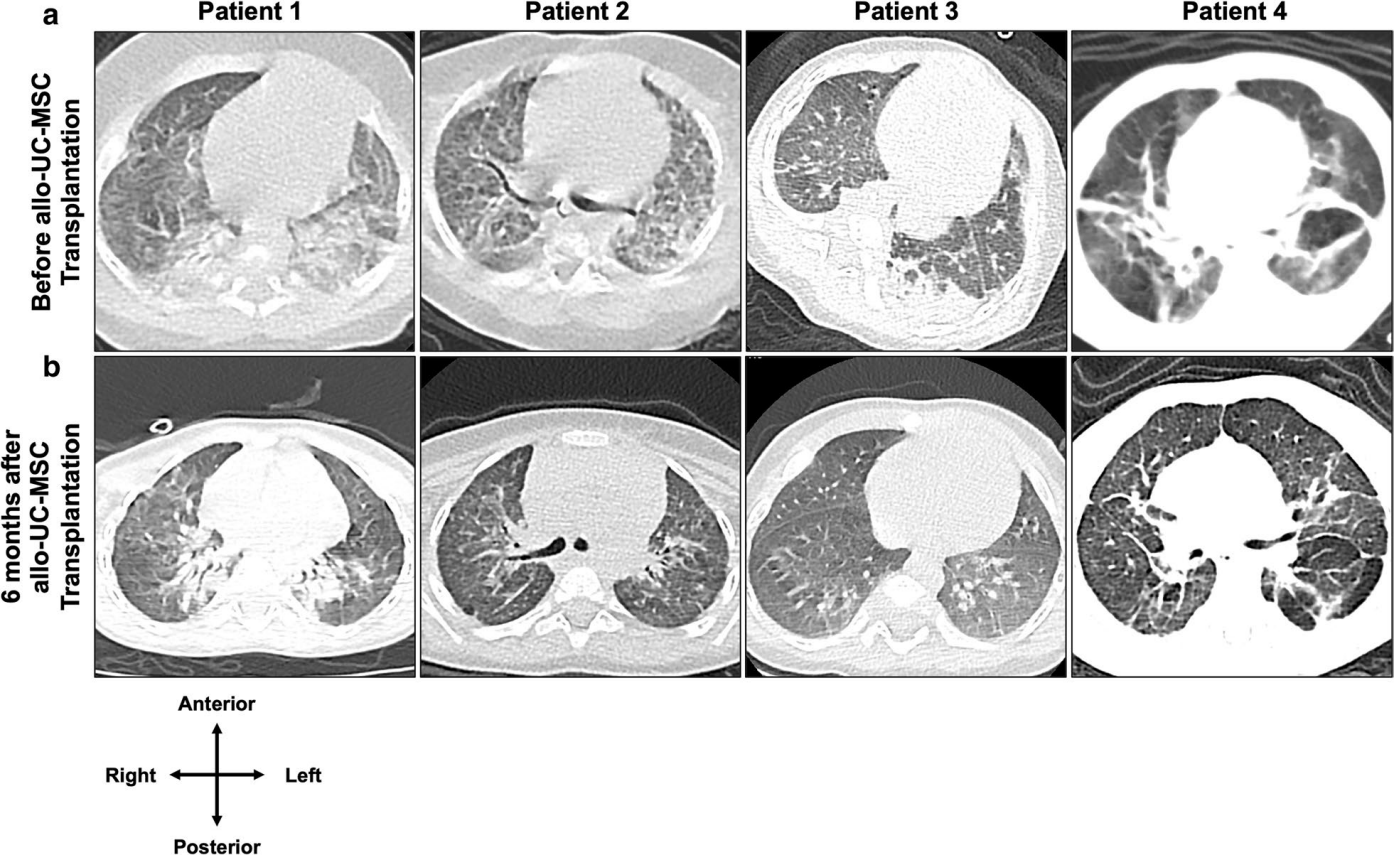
Chest CT scan indicating the recovery progression in lung structure before (a) and after (b) UC-MSC administration in all four infants with BPD
